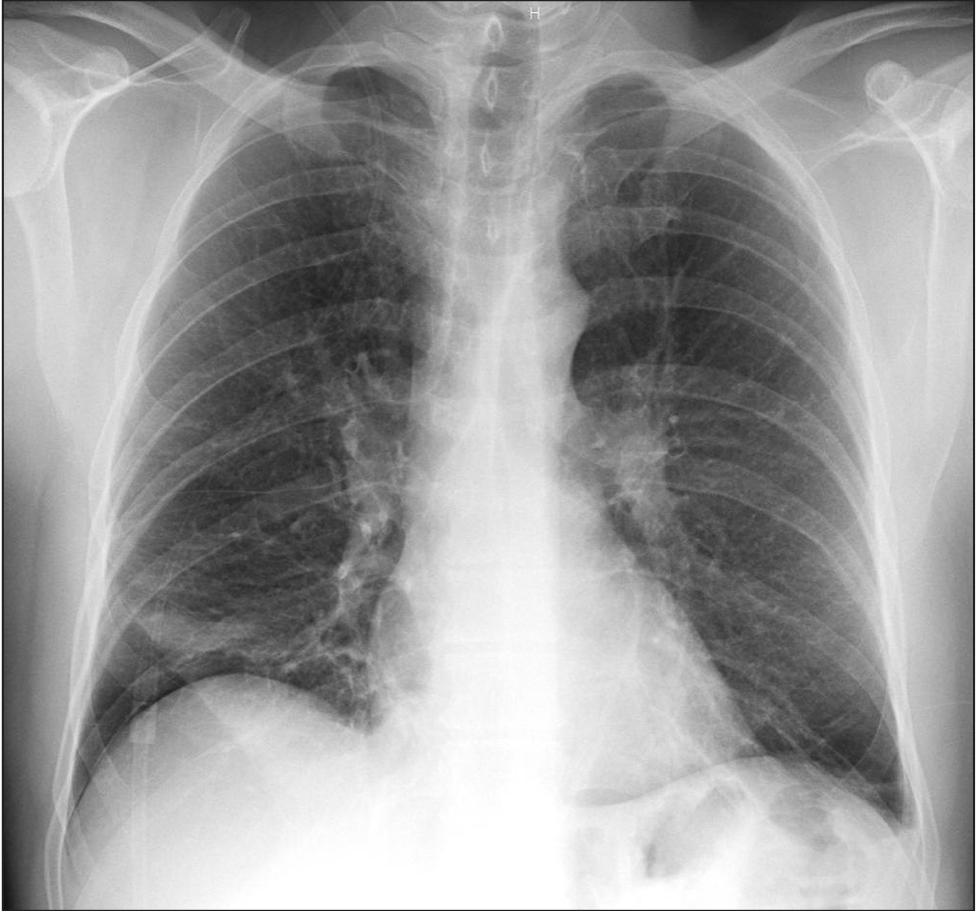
Chest X-ray (PA) showing left linear atelectasis, right subsegmental atelectasis, small left pleural effusion in a patient who presented to the emergency department with no breathing difficulty.
